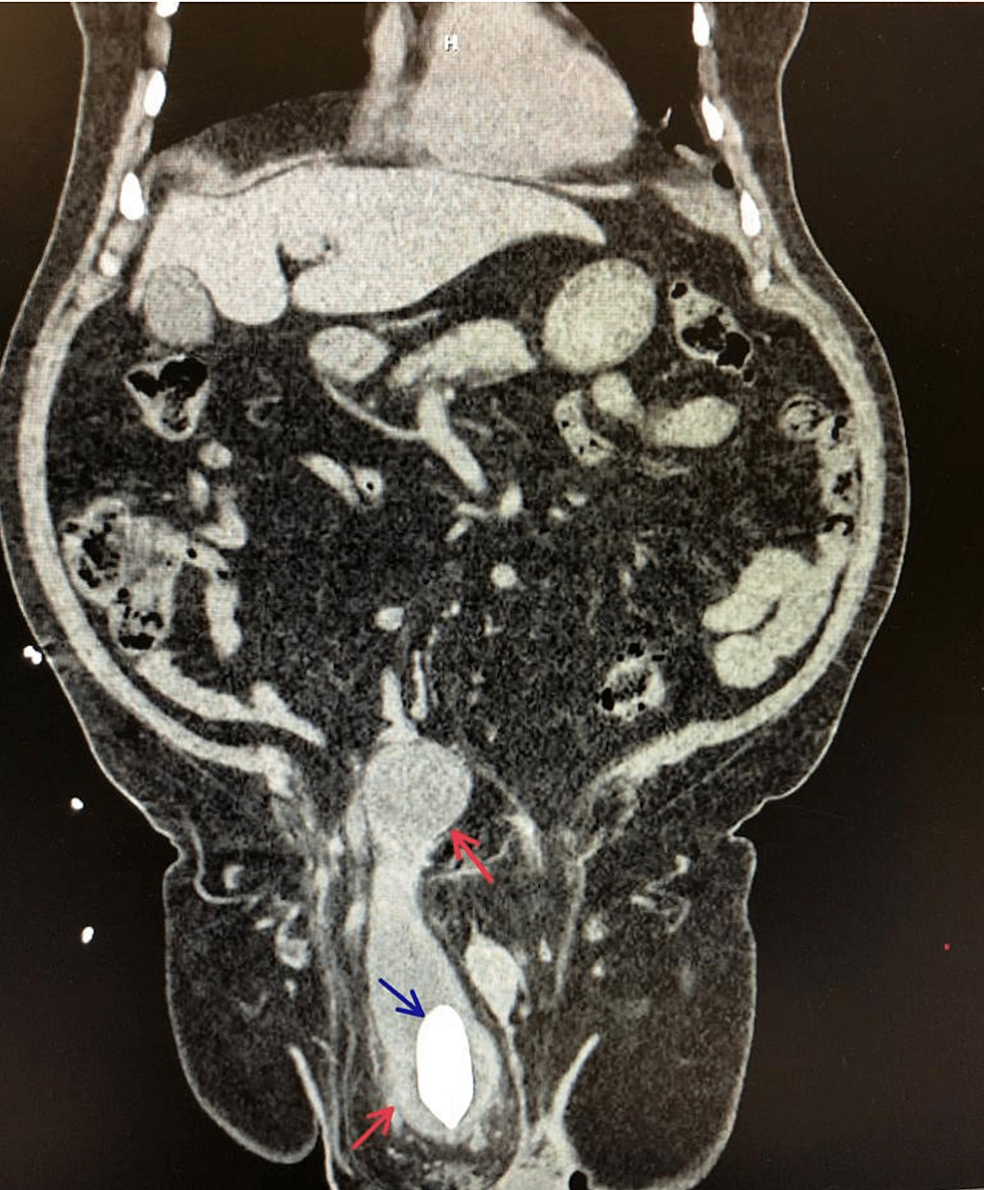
A coronal CT scan showing a pantaloon urinary bladder herniation (red arrows) with a calculus (blue arrow).
